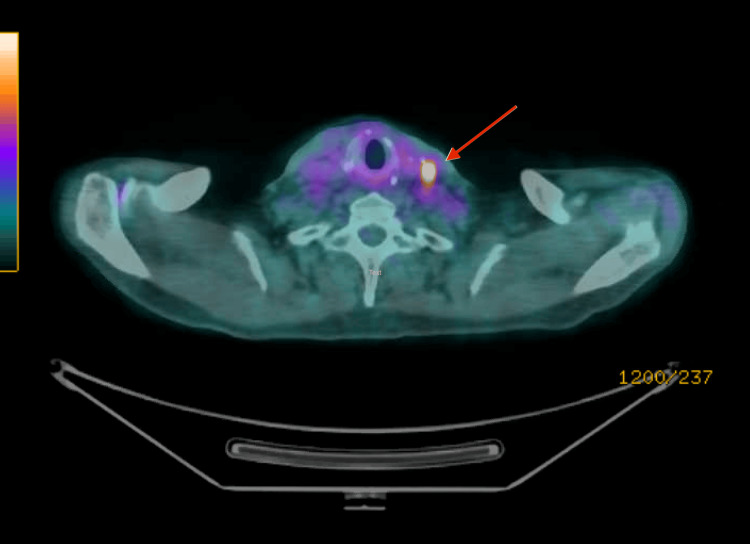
FDG-avid cervical lymphadenopathy at left level III FDG: fluorodeoxyglucose
